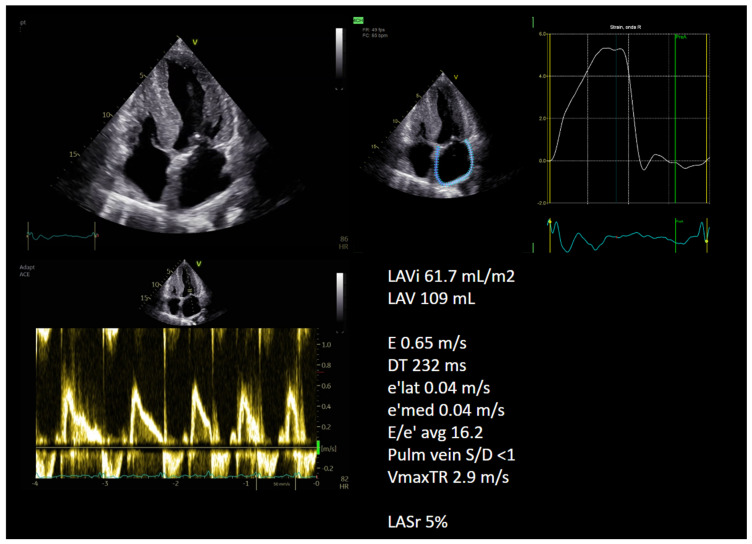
Typical echocardiographic features of a patient with transthyretin cardiac amyloidosis and atrial fibrillation: severe LA dilation and atrial wall thickening; markedly reduced left atrial reservoir strain, with absence of atrial contraction consistent with atrial fibrillation; diastolic dysfunction with indirect evidence of elevated left ventricular filling pressures. LAVi, left atrial volume indexed; LAV, left atrial volume; E, E wave; DT, deceleration time; E/e’avg, average E/e’; Pulm vein S/D, pulmonary vein systolic/diastolic ratio; VmaxTR, maximum tricuspid regurgitation velocity; LASr, Left Atrial Reservoir Strain.
